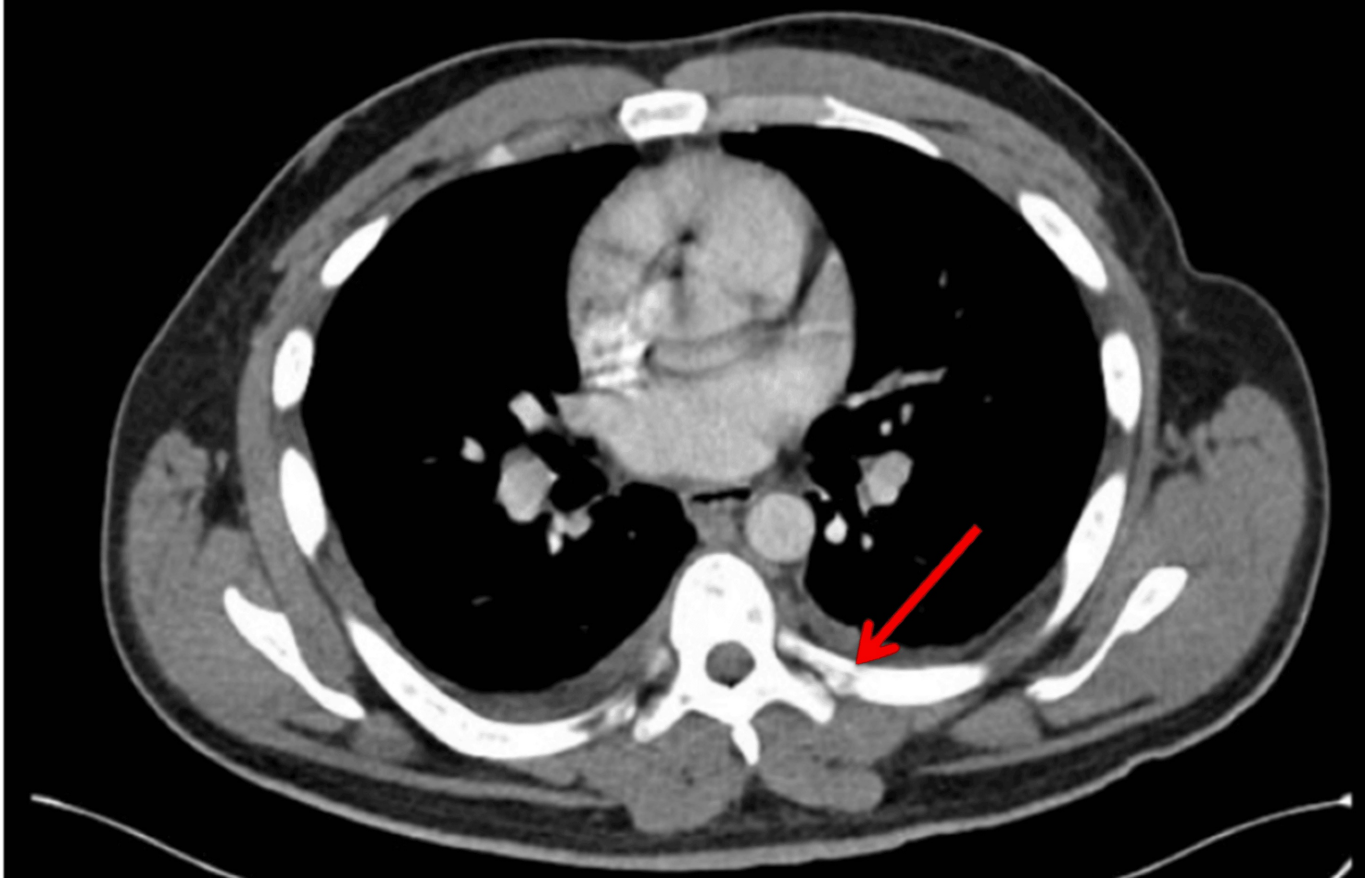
CT scan showing a sclerotic lesion with cortical breakdown in the left posterior sixth rib (red arrow)
